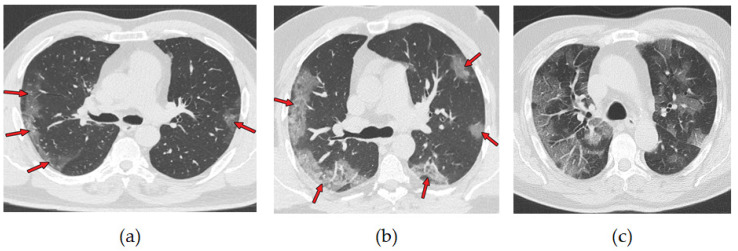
Axial nonenhanced chest CT images (lung window) in a 59-year-old man (a) and a 47-year-old man (b) show bilateral areas of ground-glass opacities (arrows) in a peripheral distribution; (c) shows bilateral ground-glass opacities and dilated segmental and subsegmental vessels, mainly on the right, in a 70-year-old man, each with positive RT-PCR test results for SARS-CoV-2. Adapted from [10].
